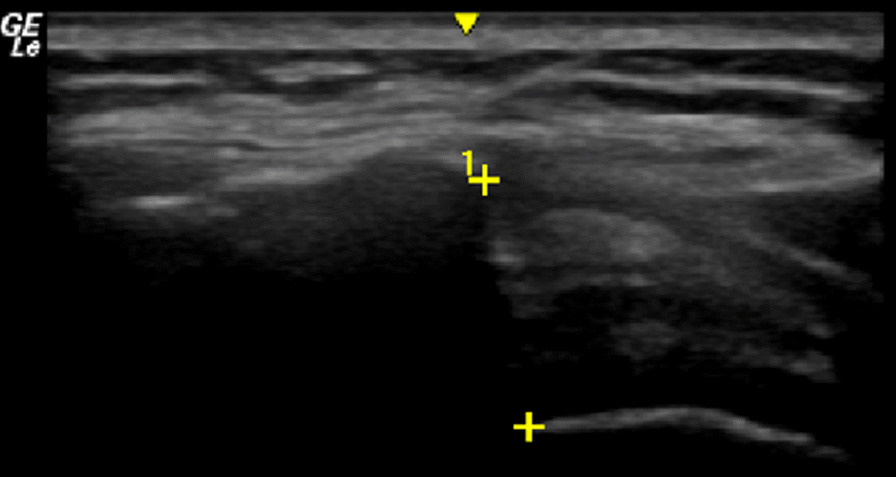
Ultrasound image of the subacromial space. Crosses (+) show the superior (acromion) and inferior (humerus) landmarks of the subacromial space
